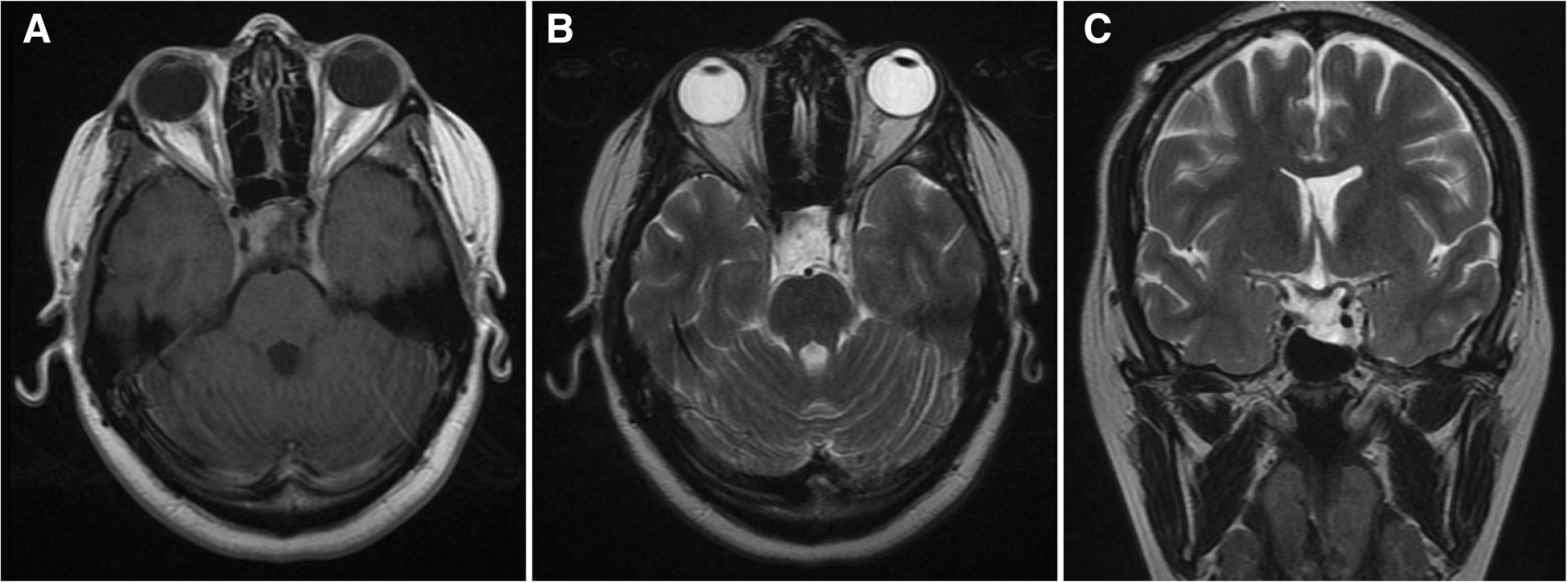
Last pituitary magnetic resonance imaging performed in September 2017. Pituitary tumor (20 × 15 × 20 mm) in axial T1-weighted fluid-attenuated inversion recovery (a), axial T2 (b) and coronal T2 images (c)
